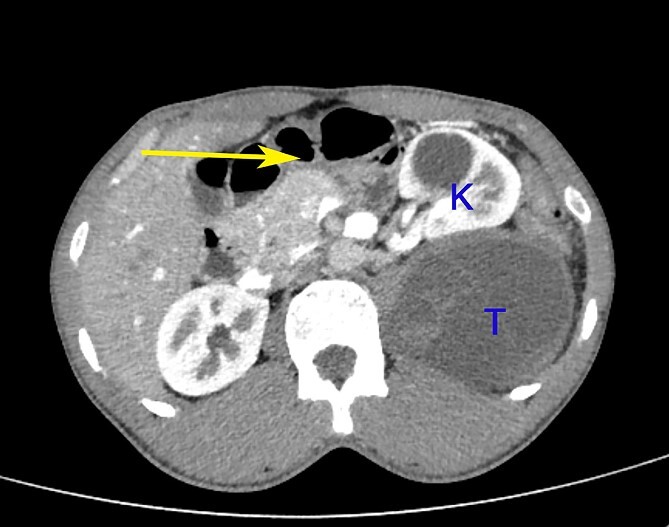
A contrast-enhanced CT scan of the abdomen at the level of the L2 vertebra shows a paravertebral tumour (T) posterior to the kidney (K). The tumour shows peripheral enhancement and inhomogeneous intra-tumoural contrast enhancement. The left colon is pushed to the right and anteriorly (arrow).
